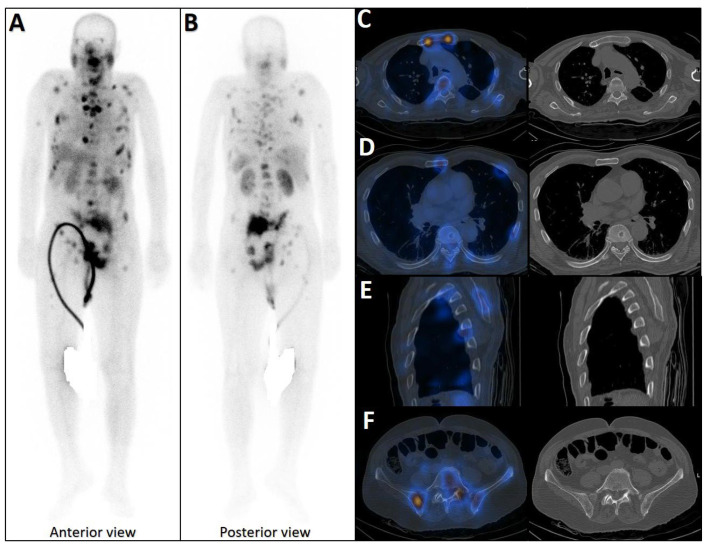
Early phase of metastases without clear sclerotic changes in the bone. (A) 70-year-old man with elevated PSA was recently diagnosed with prostate adenocarcinoma (all cores positive with Gleason score 5 + 4) and referred for staging. The whole-body 99mTc-PSMA scan (A,B) and SPECT/CT showed multiple PSMA-avid skeletal metastases, most of which showing no/minimal density changes (C–F).
